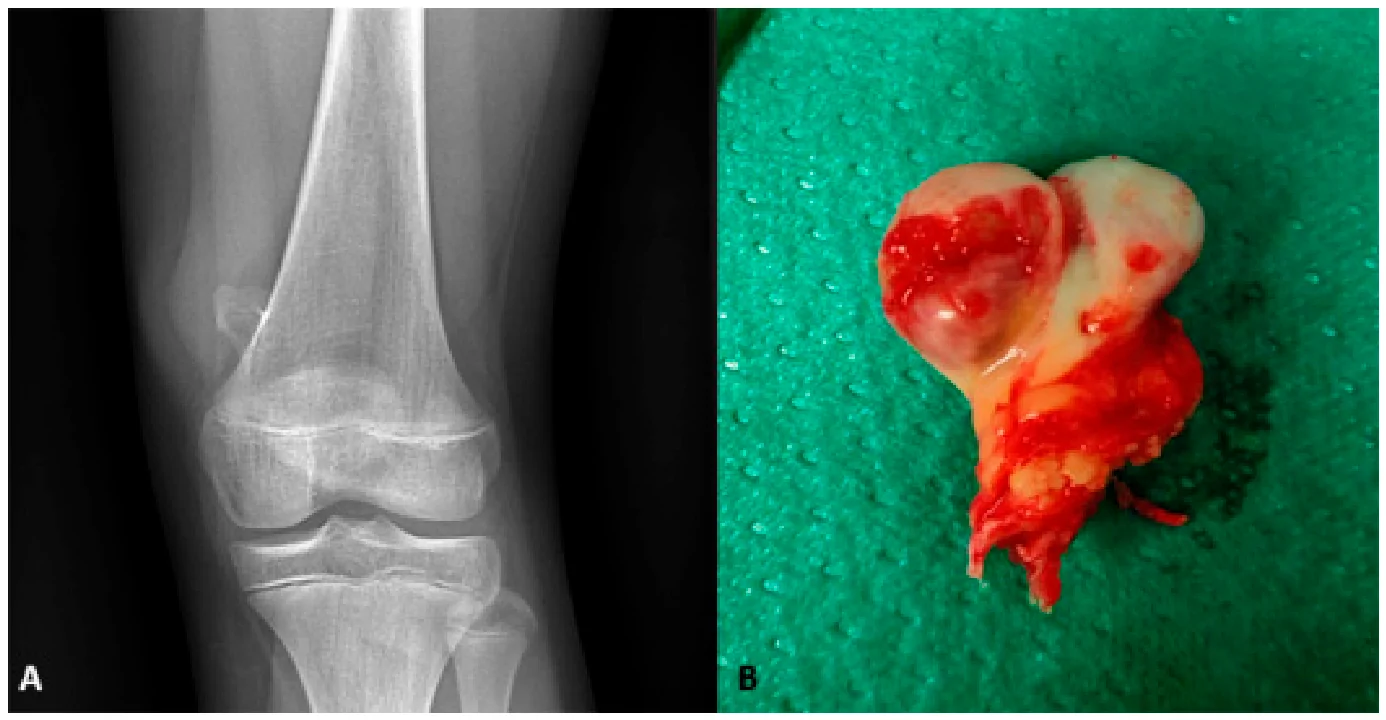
Osteochondroma of the distal femur in a 14-year-old male adolescent. (A) Preoperative radiograph demonstrating a well-defined exophytic bone lesion arising from the distal femur; (B) Intraoperative view showing the lesion after surgical exposure and complete excision.
